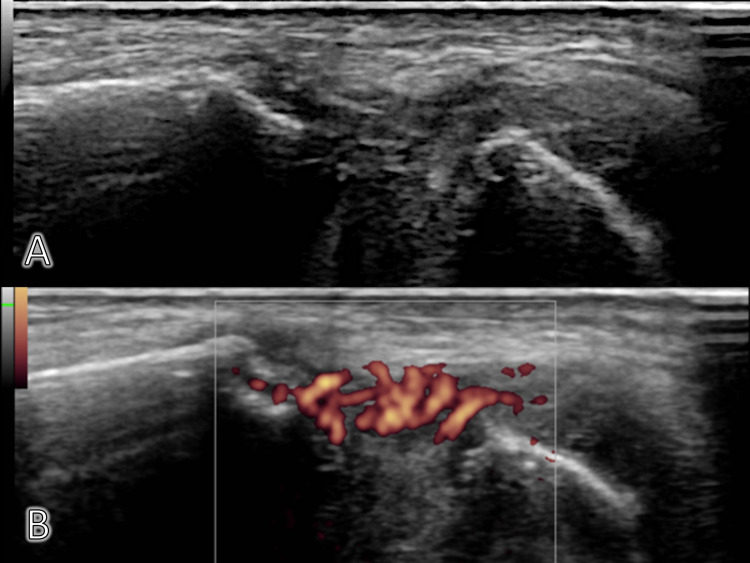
Ultrasound images of the left sternoclavicular joint The image shows fine flow in the joint space (A: Plain, B: With fine flow)
